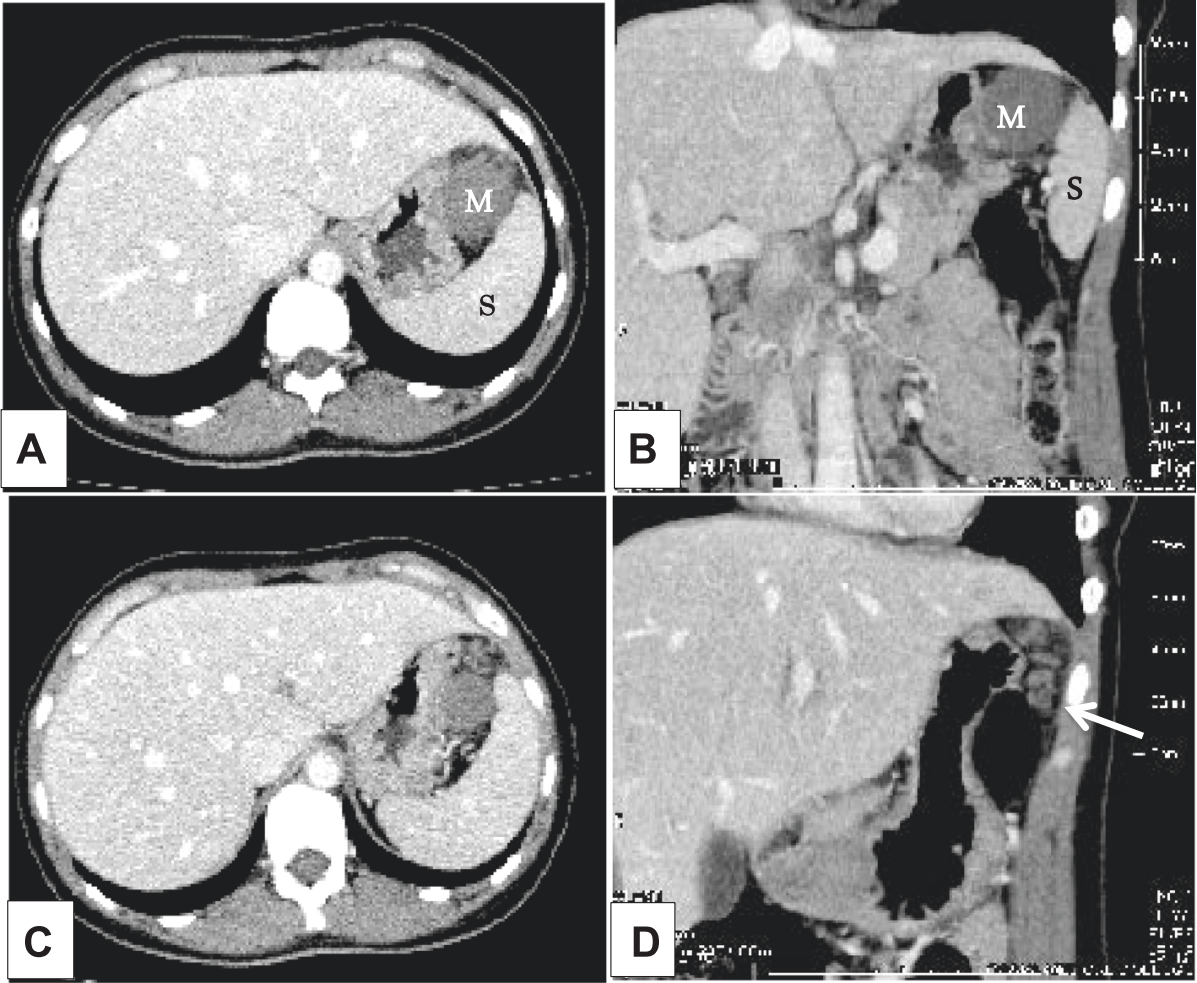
Axial and coronal contrast-enhanced CT images (a axial, b coronal). This study showed a normally enhanced spleen (S) in left upper quadrant and a non-enhancing mass (M) anterior to the spleen (c axial, d coronal). The twisted tubular structure (*arrow*) attached to the mass was detected
